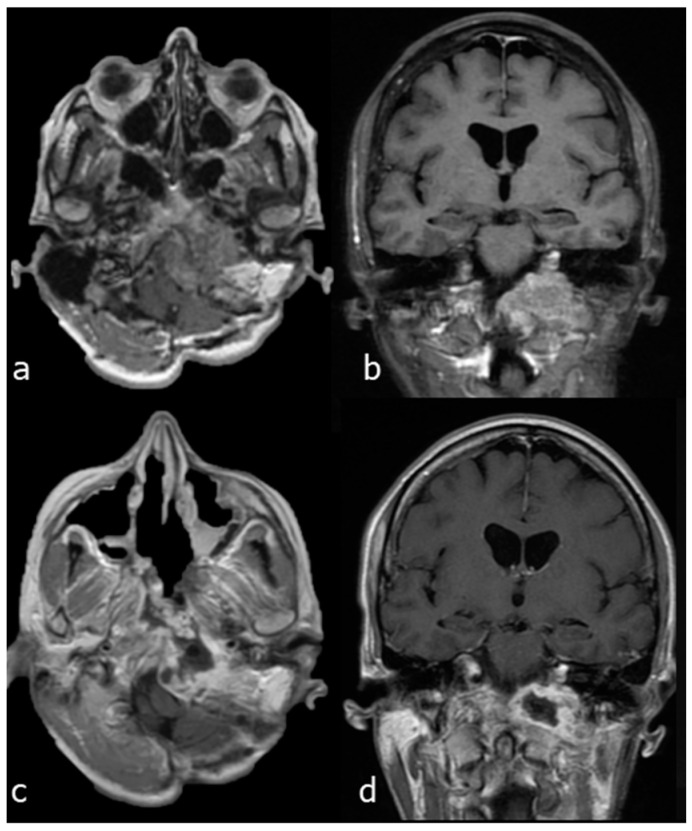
Case 1: preoperative and postoperative MRI scans of a large cranio-cervical junction chordoma, underwent first surgery through a retrosigmoid craniotomy, and then operated on by a combined EETC and CTM approach. (a) preoperative MRI scan, axial view. (b) preoperative MRI scan, coronal view. (c) postoperative MRI scan, axial view. (d) postoperative MRI scan, coronal view.
